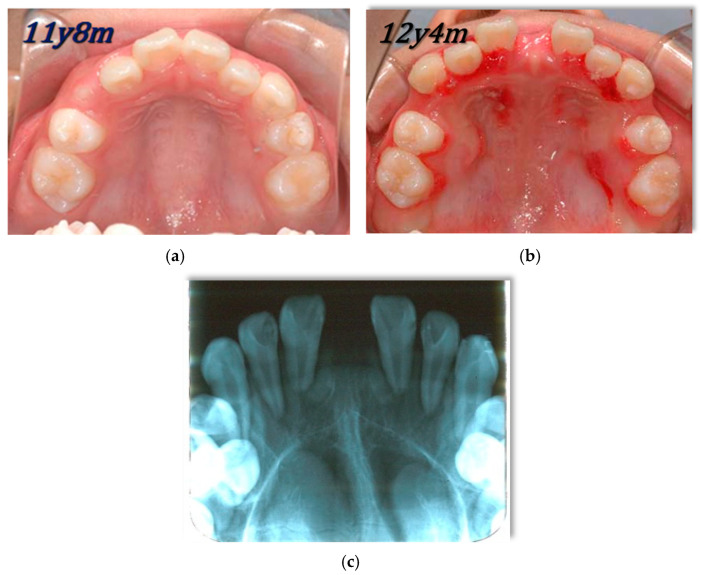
A representative case where a prominent anterior diastema between the central incisors developed following therapy; (a) maxillary occlusal plane before sRME, (b) maxillary occlusal plane after sRME, (c) X-ray photo of maxillary occlusal plane after sRME.
